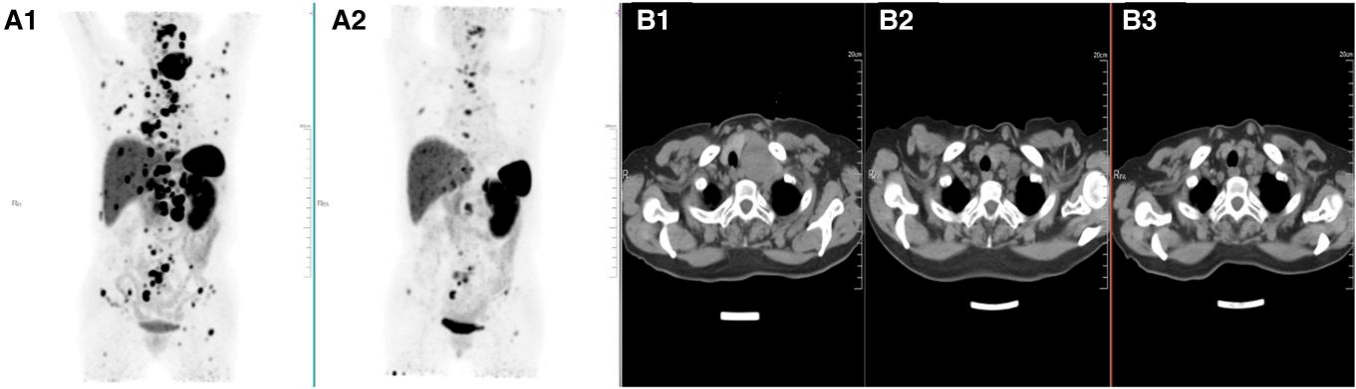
Baseline and review imaging in an anlotinib-treated female patient (aged 57 years old) with retroperitoneal paraganglioma accompanied by metastases in liver, bone, breast, and multiple lymph node. (A1, A2) Maximum intensity projection of 68Ga-DOTA-NOC PET/CT of baseline images and images after 8 months treatment; (B1-B3) CT images of metastases of left cervical lymph node of images on baseline, after 4 months treatment, after 8 months treatment.
